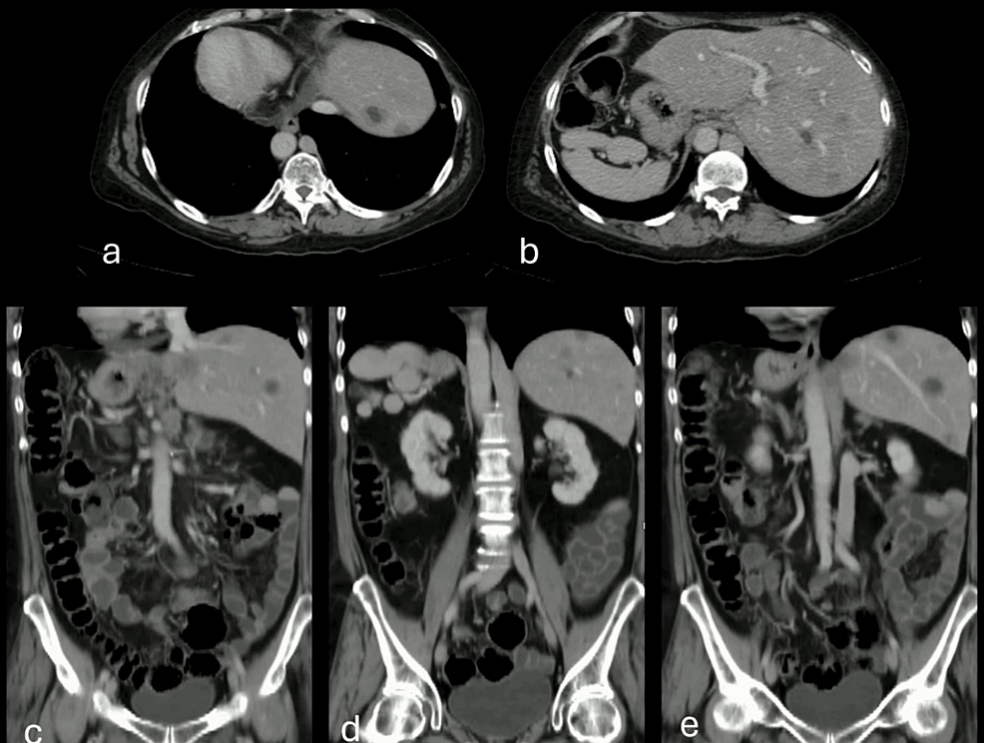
Detailed CT images of the patient. (a) The axial CT image shows the heart on the right side, indicating dextrocardia. (b) The axial image shows the liver on the left and the spleen, with accessory spleens on the right. (c) The coronal reconstruction image shows multiple well-defined liver lesions with peripheral enhancement and necrotic centers, post-Whipple surgery changes, and an absent hepatic IVC segment continuing into the azygos system and dextrocardia. (d) The coronal reconstruction shows polysplenia and abdominal situs inversus. (e) The reconstruction shows the colon on the right and the jejunum and ileum on the left, indicating intestinal malrotation. IVC: inferior vena cava
